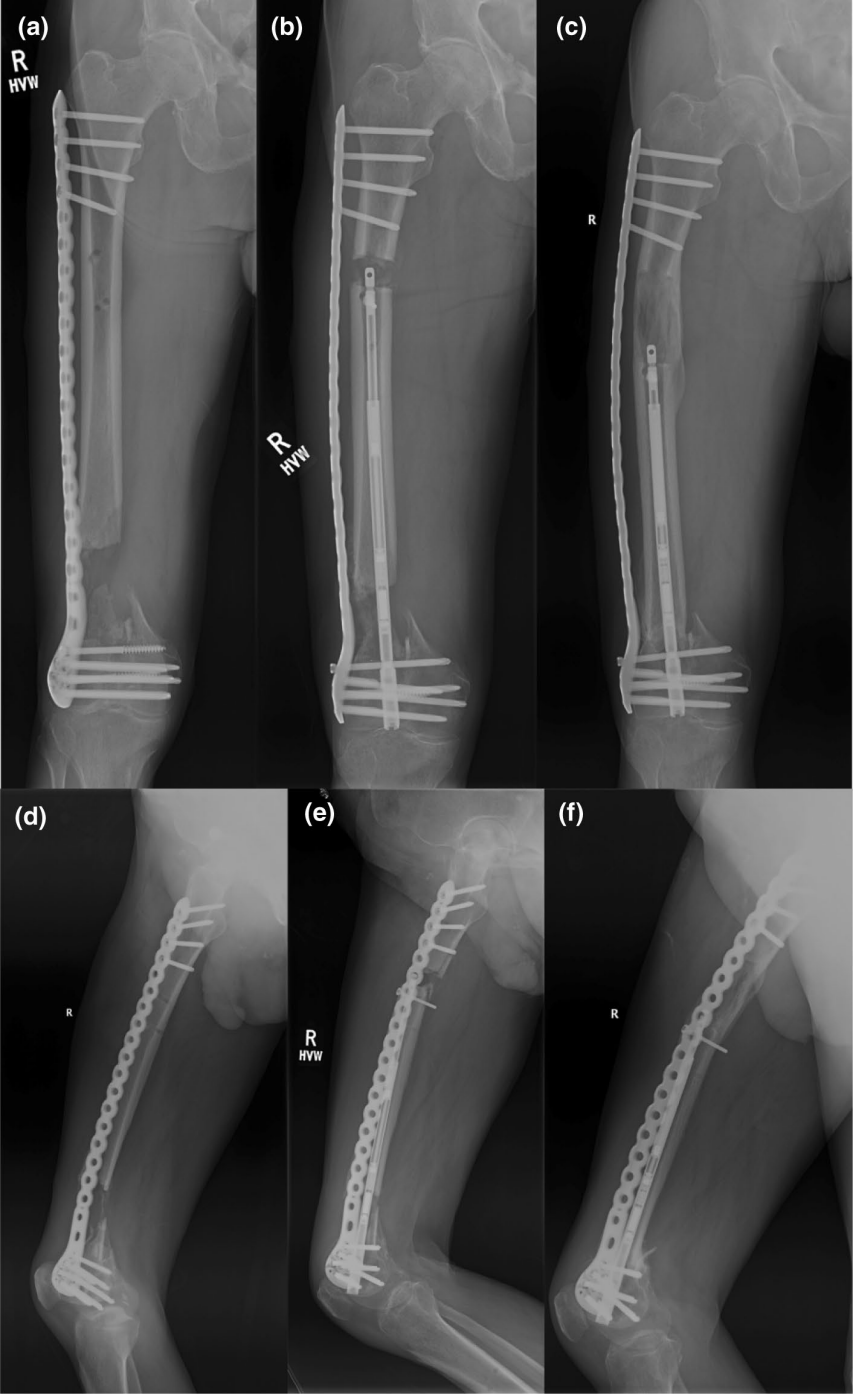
AP (a, b, c) and lateral (d, e, f) radiographs of Patient 4 showing the defect at the start, during the retrograde bone transport and at the final stage
